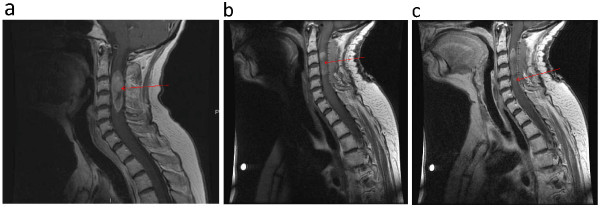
Sagittal cervical spine MRI. (a) Pre-surgical resection, T1 post-contrast demonstrating a 1.3 × 4.4 cm intramedullary enhancing mass (left panel). (b) Post-surgical resection, T1 pre-contrast (middle panel). (c) Post-surgical resection, T1 post-contrast showing small amount of residual tumor at caudal margin of tumor (right panel).
